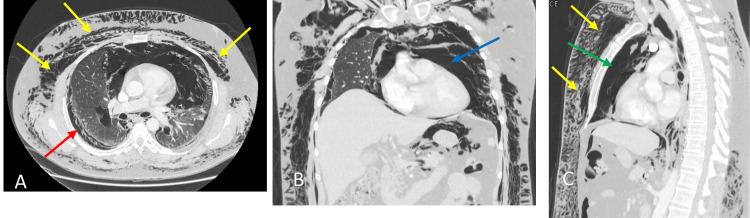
Axial (A), coronal (B), and sagittal (C) views of CT thorax demonstrating subcutaneous emphysema (yellow arrows), pneumothorax (red arrow), pneumopericardium (blue arrow), and pneumomediastinum (green arrow).
